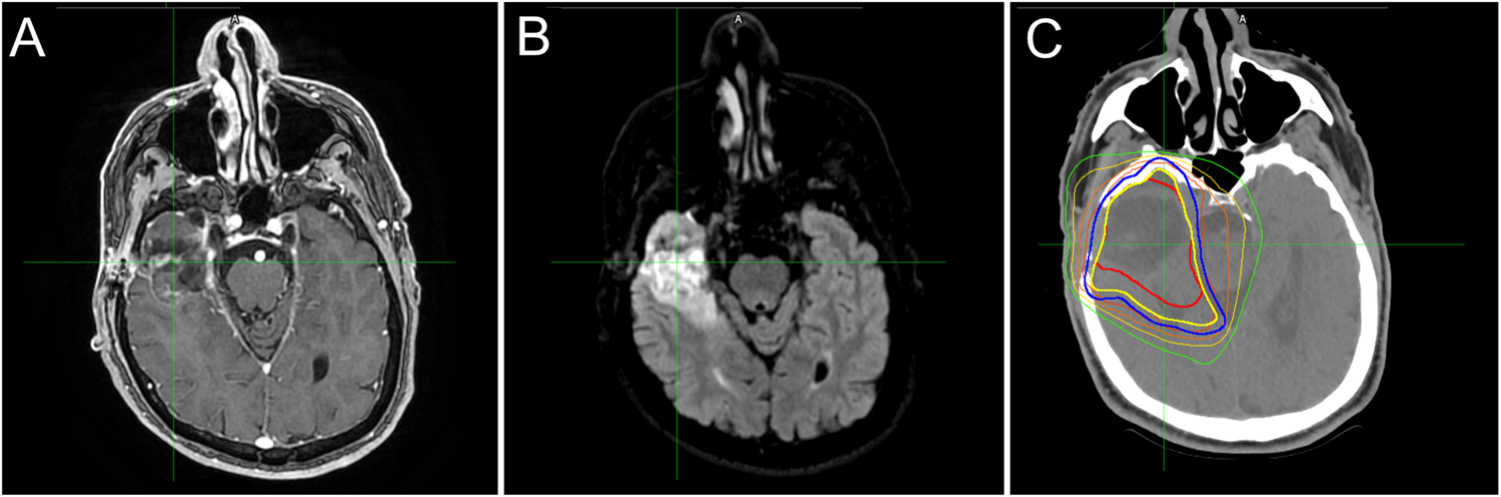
Contrast-enhanced T1w (A) and FLAIR-weighted (B) images, obtained after surgery of a lesion consistent with a glioblastoma, demonstrate the surgical cavity with peripheral signal abnormality, which is included in the distribution of doses of the centering CT (C)
